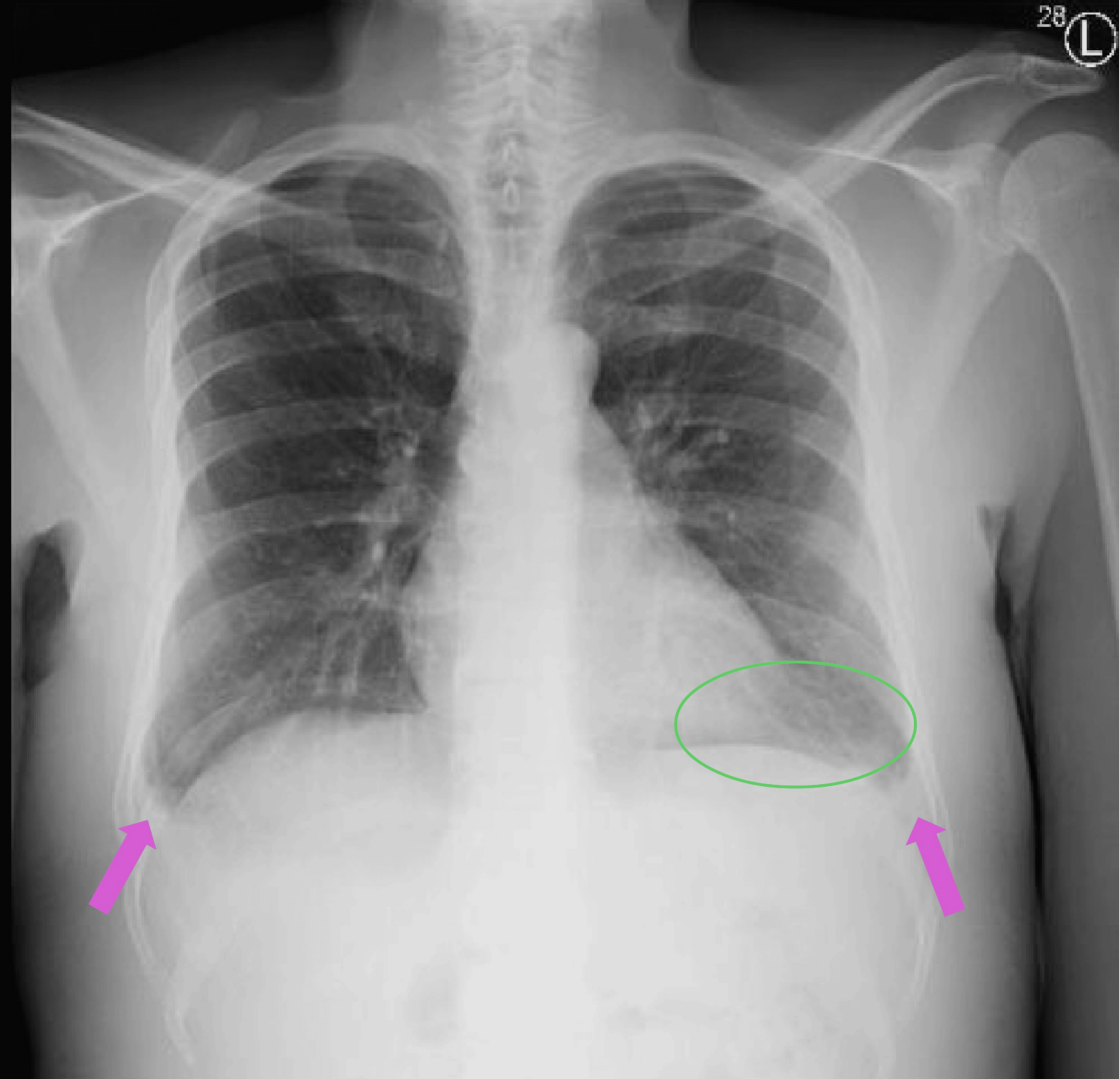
Chest X-ray Chest radiograph showing no evidence of pneumonia. Also seen are discoid atelectasis in the left lung base (green circle) and blunting of the costophrenic angles (pink arrows), possibly due to minimal effusion. The remaining lung fields show no active infiltrates or consolidations.
